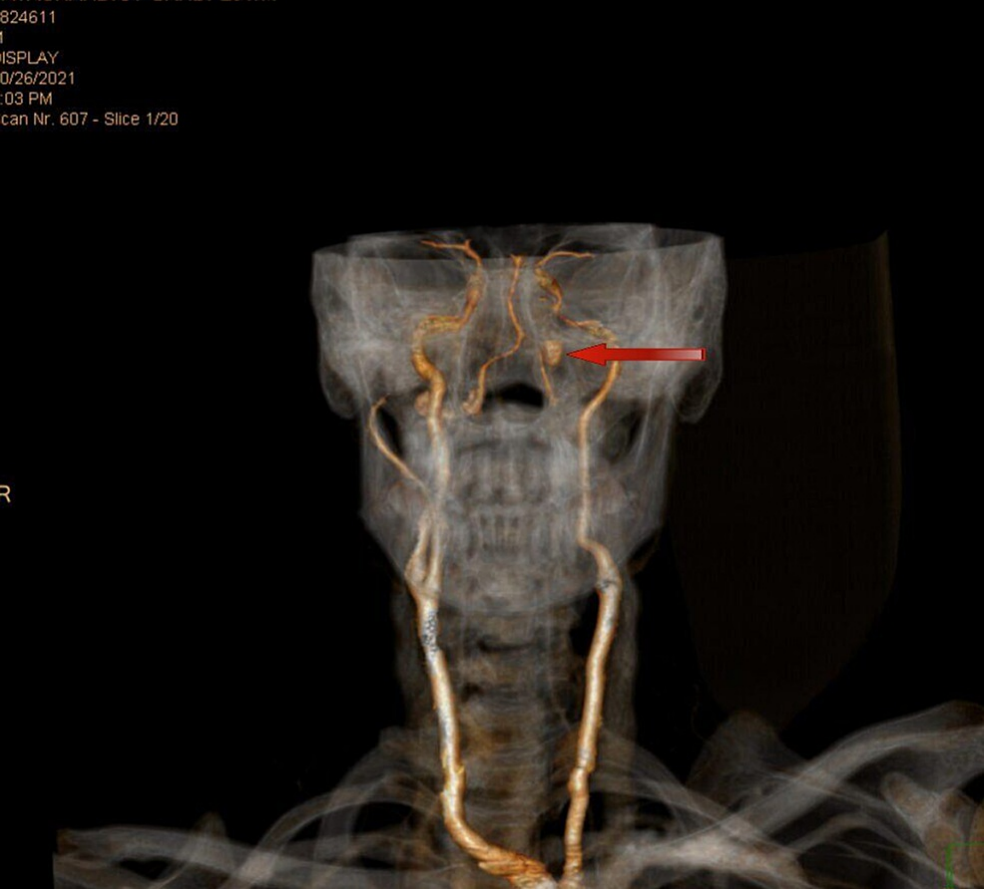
CT angiogram anterior view demonstrating a thin-walled aneurysm (red arrow) in the cavernous segment of the left ICA. CT: computerized tomography; ICA: internal carotid artery
